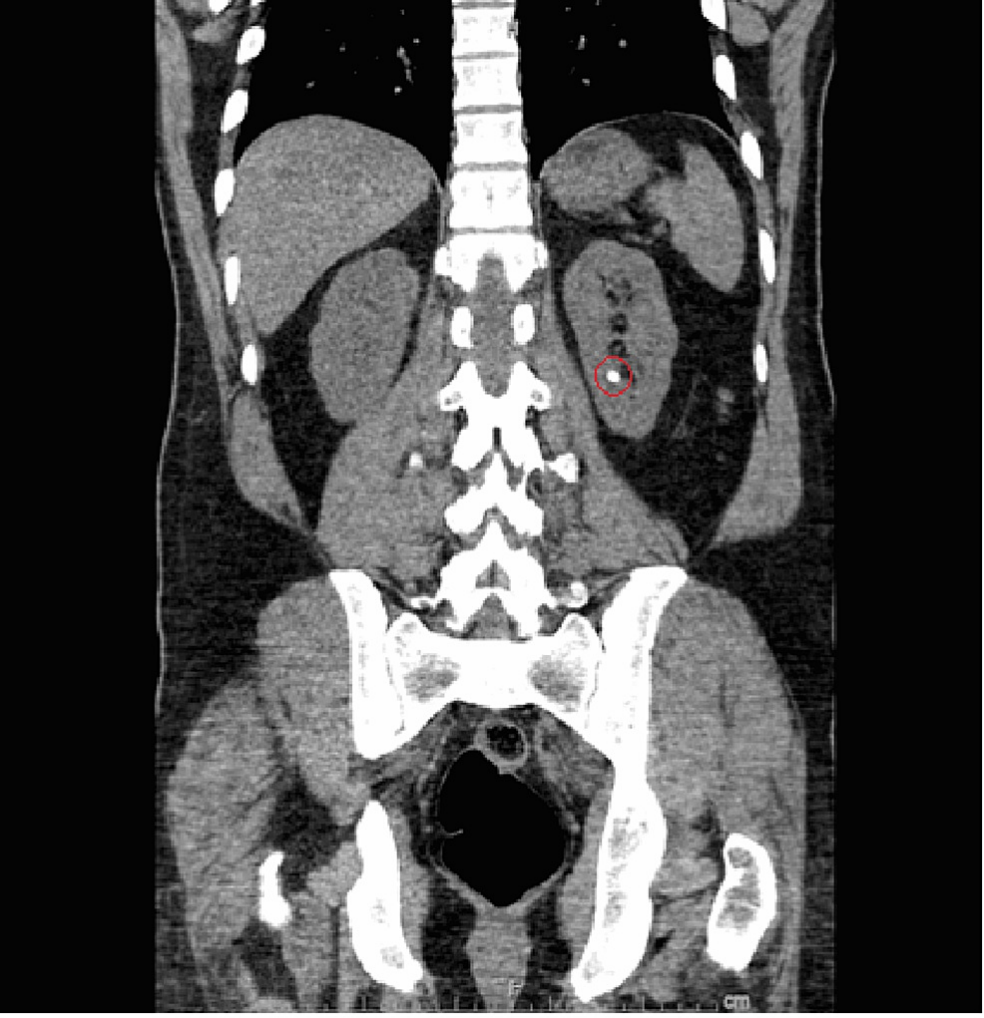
Coronal view of CT abdomen pelvis without IV contrast. Red circle marking 6 mm non-obstructing left renal stone.
